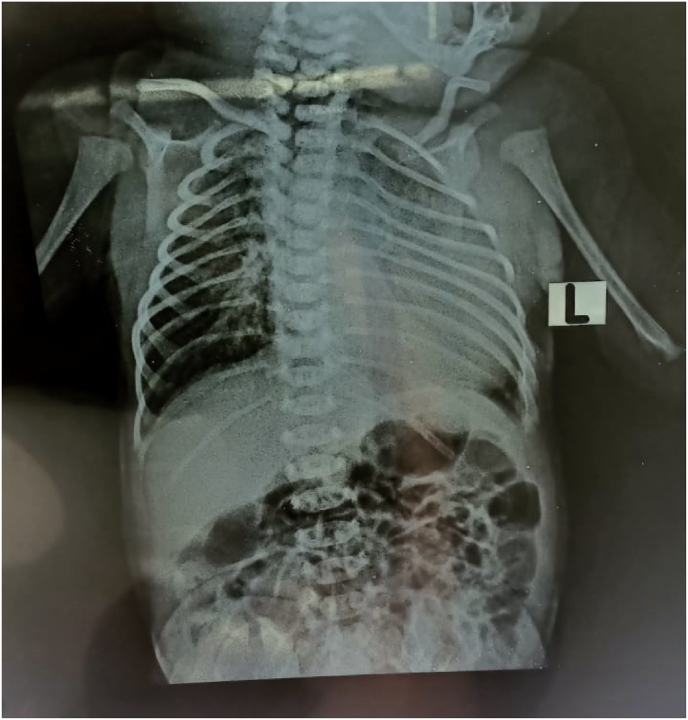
A section of the chest radiograph, X-ray findings revealed ….
